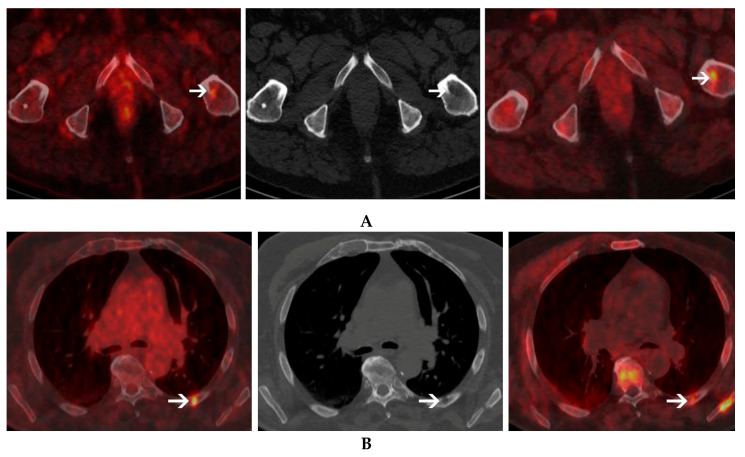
A 72-year-old male with light chain multiple myeloma. ^18^FDG PET/CT (left panel) and ^18^F-Choline PET/CT (right panel) were performed with a 5-day-interval. Different uptake patterns are seen in this patient. Lesion exhibiting high choline uptake but low FDG uptake can be seen in the left femur (A). In contrast, however, a lesion with high FDG uptake but low Choline uptake can be seen in the posterior arch of the left 6th rib (B) corresponding to an osteolytic lesion on CT (central panel).
